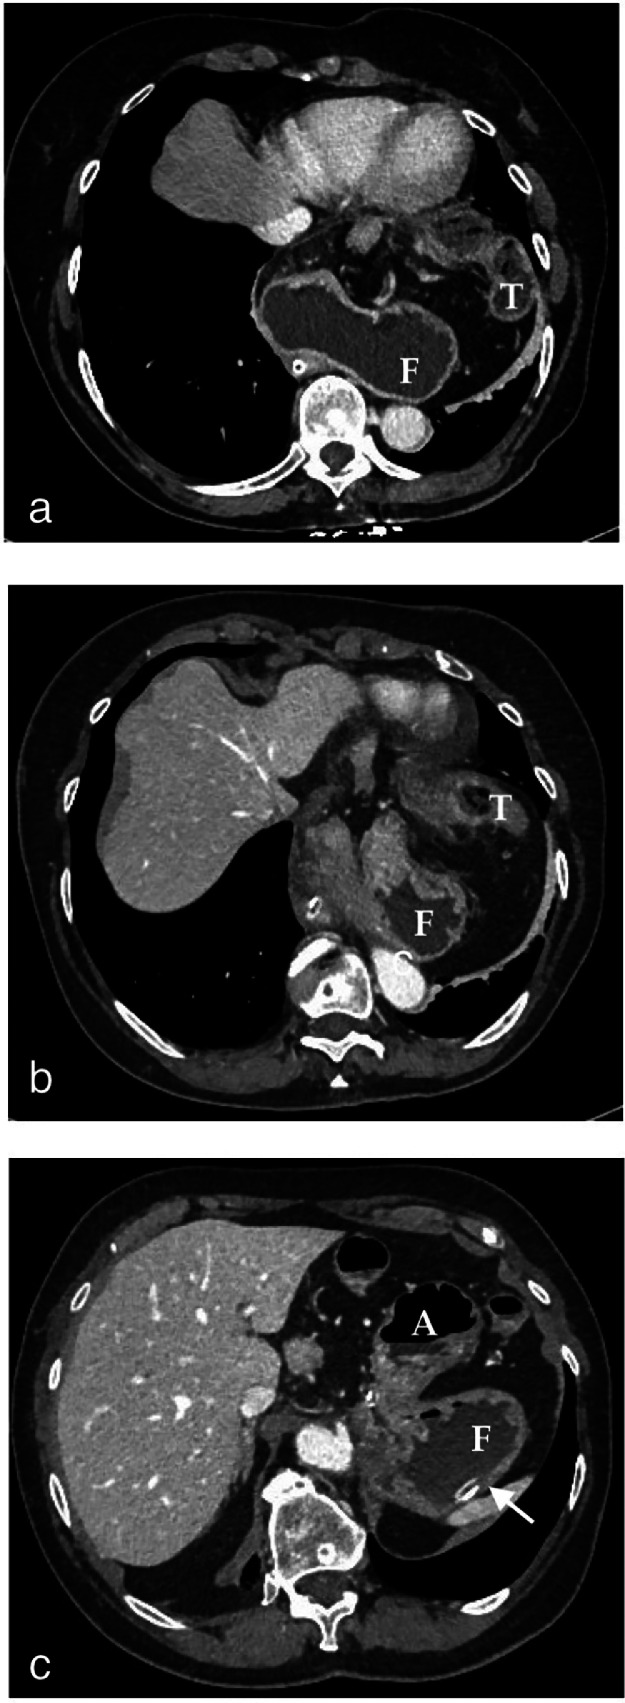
(A-C) Axial contrast enhanced CT with intravenous contrast showing a left large hiatal hernia. The transverse colon (T) is also detected within the hernial sac. The stomach appeared folded on itself with the antrum (A) at the same level and over the fundus(F). Nasogastric tube is visible at the fundus (white arrow).
